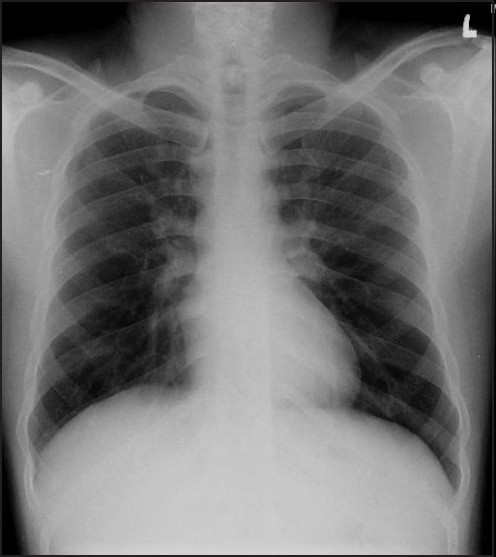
Pre-operative normal chest X-ray
